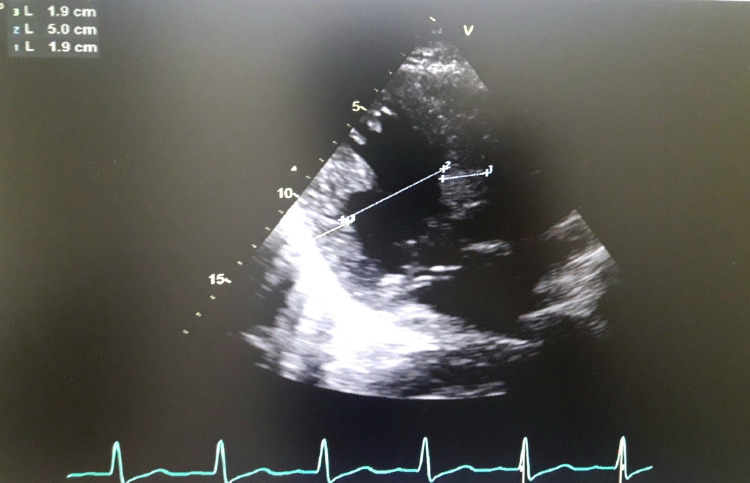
Echocardiography showing concentric hypertrophy
